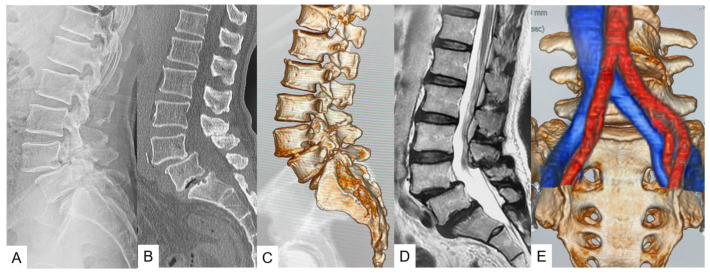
Preoperative images. (A) Lateral lumbar neutral radiogram, (B) mid-sagittal reconstruction CT, (C) 3-D CT, (D) T2-weighted mid-sagittal MR imaging, (E) Ct-MRI fusion image. For oblique lateral interbody fusion at L5-S1 (OLIF51), evaluation of the vascular window is very important.
